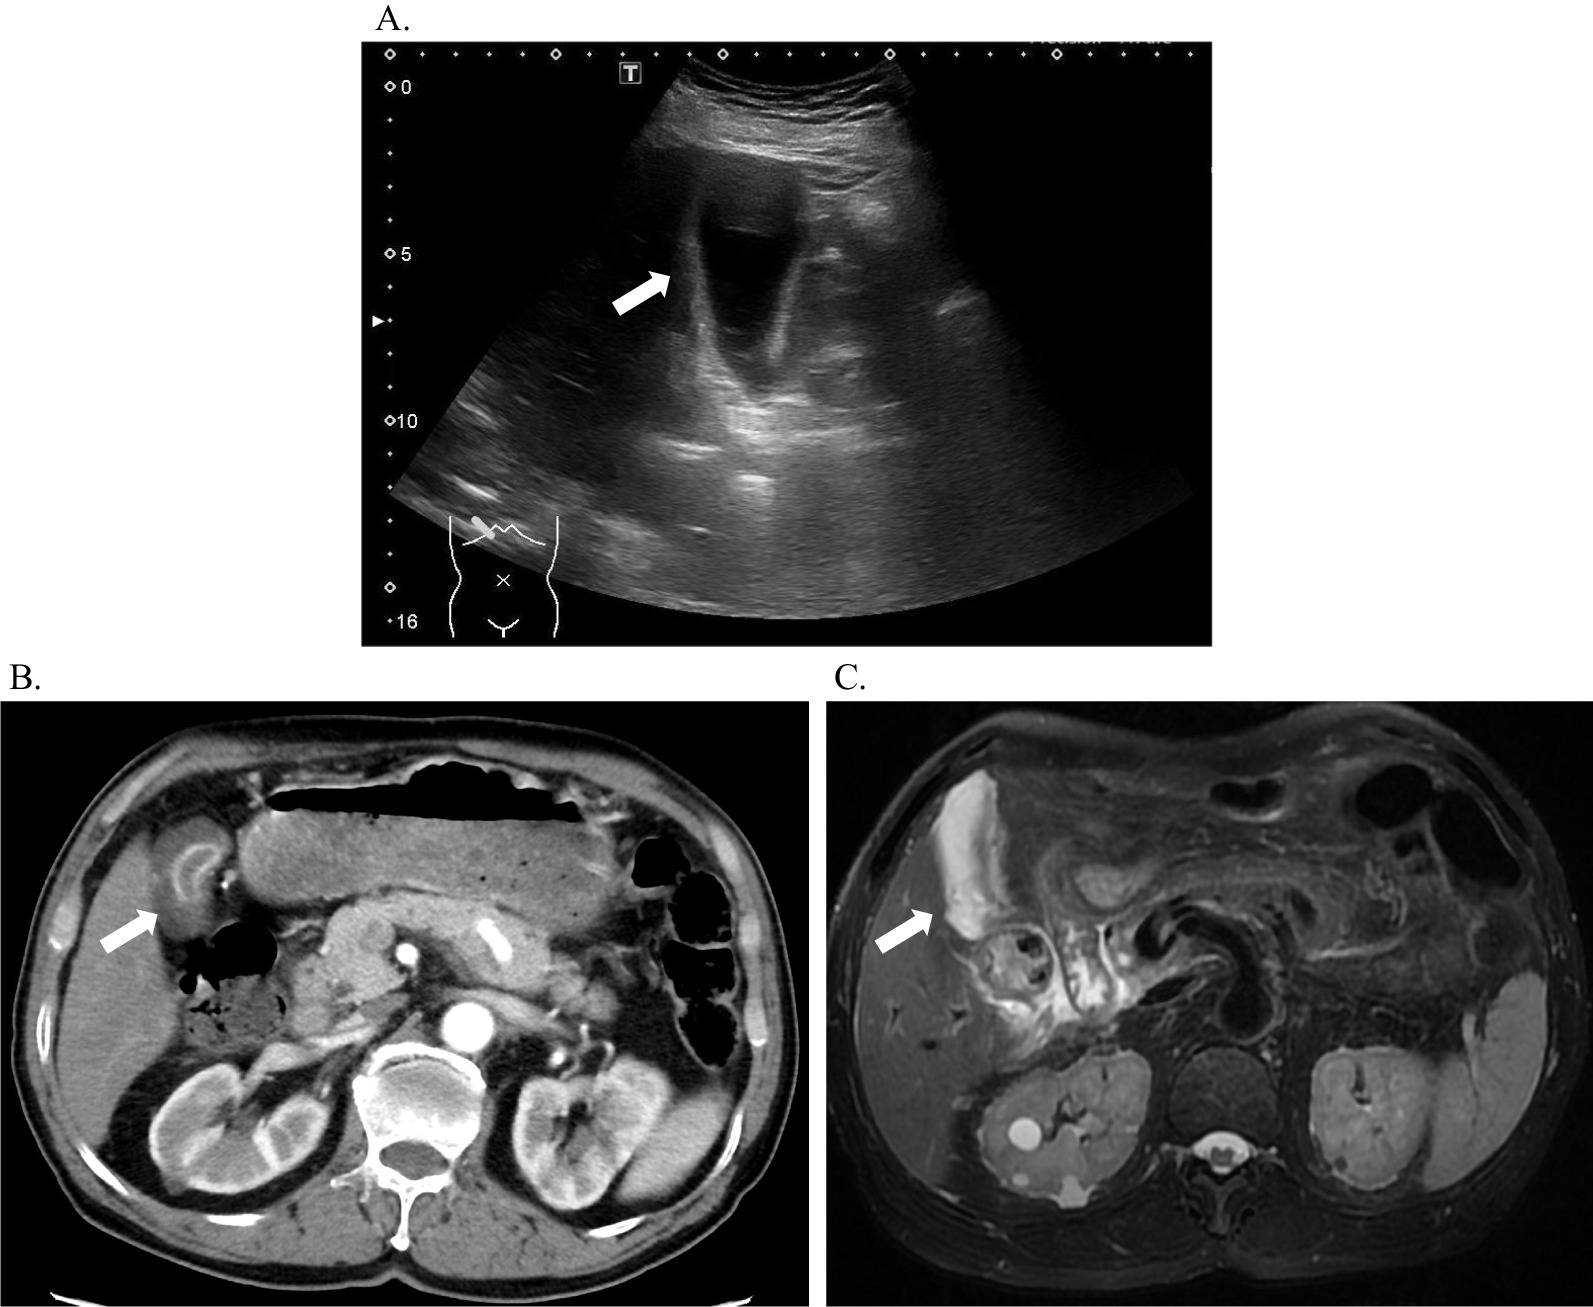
Images of gallbladder in two patients with acute hepatitis E. Abdominal ultrasound (a) showed significant thickening of the gallbladder wall (white arrow) in case 1. Both CT (b) and MRI (c) showed the presence of acalculous cholecystitis (white arrow) in case 2
